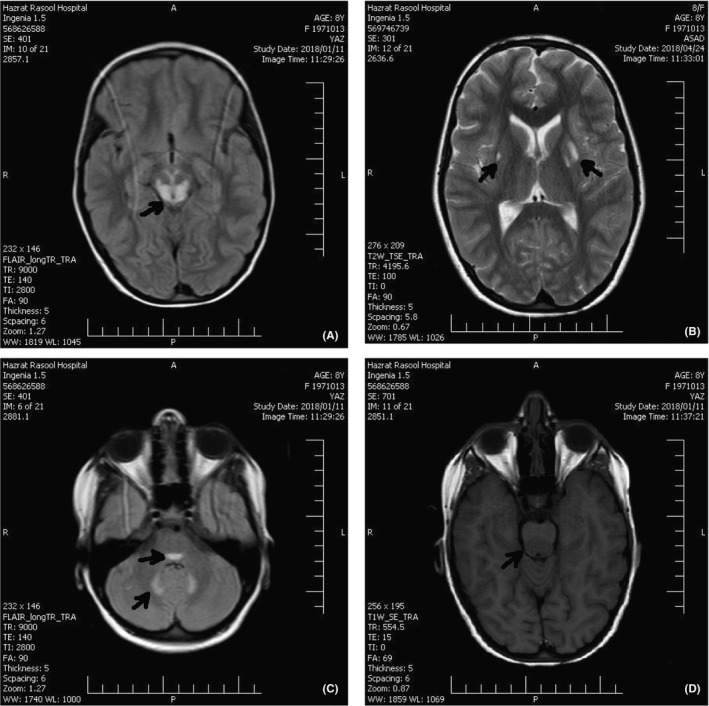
The second brain MRI. (A) Axial brain MRI (flair): Arrow shows hyperintensity in dorsal midbrain; (B) Axial brain MRI (T2): Arrows show bilateral hyperintensity in putamen nucleus; (C) Axial brain MRI (flair): Arrows show hyperintensity in dorsal midbrain and dentate nucleus of the cerebellum; (D) Axial brain MRI (T1): Arrow shows hypointensity in dorsal midbrain area
